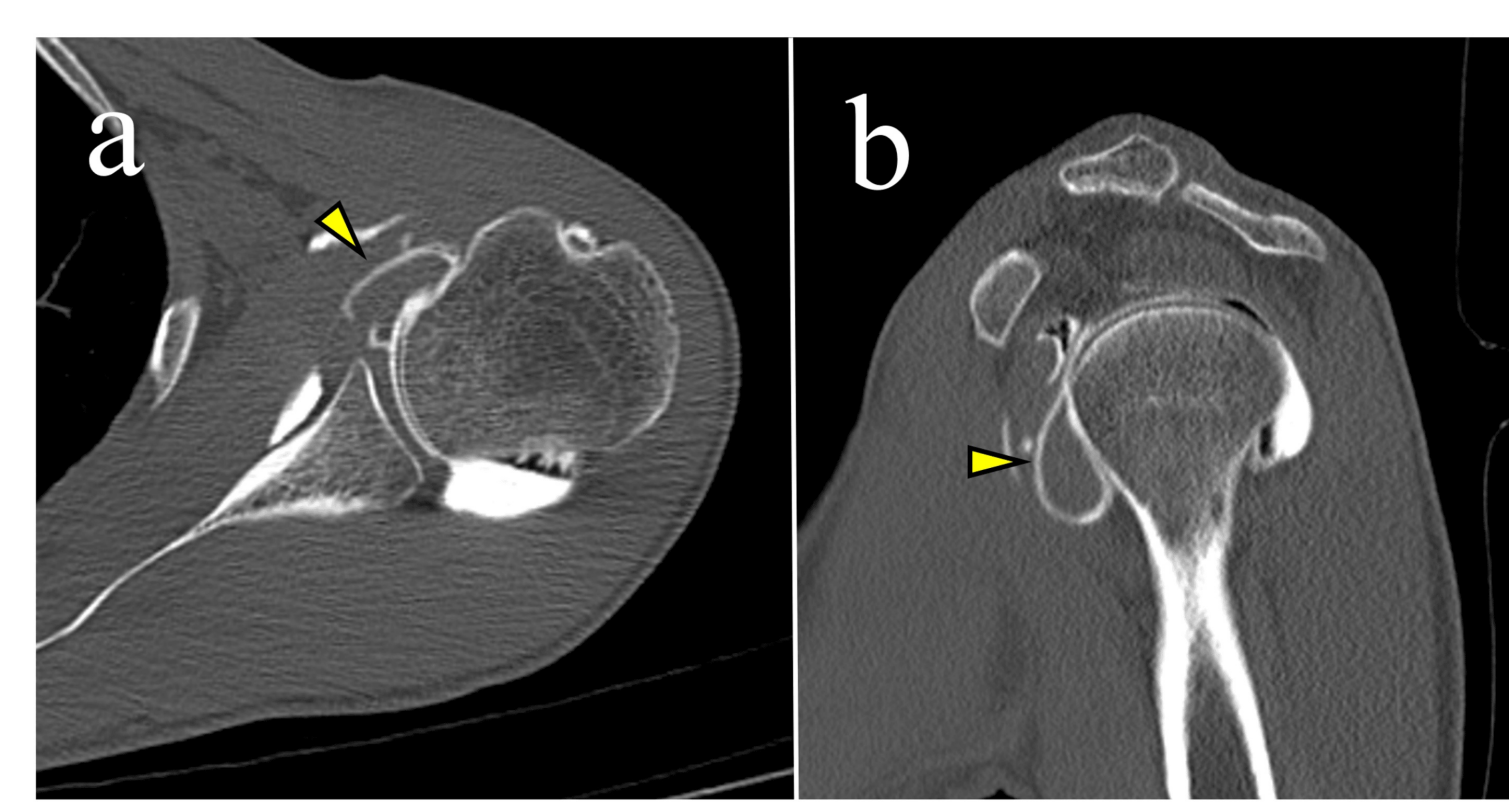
Intra-articular contrast-enhanced computed tomography obtained six years before referral to our institution (a) Axial and (b) sagittal images demonstrating an anterior intra-articular contrast filling defect (arrowheads) with peripheral contrast enhancement, suggestive of an intra-articular mass.
